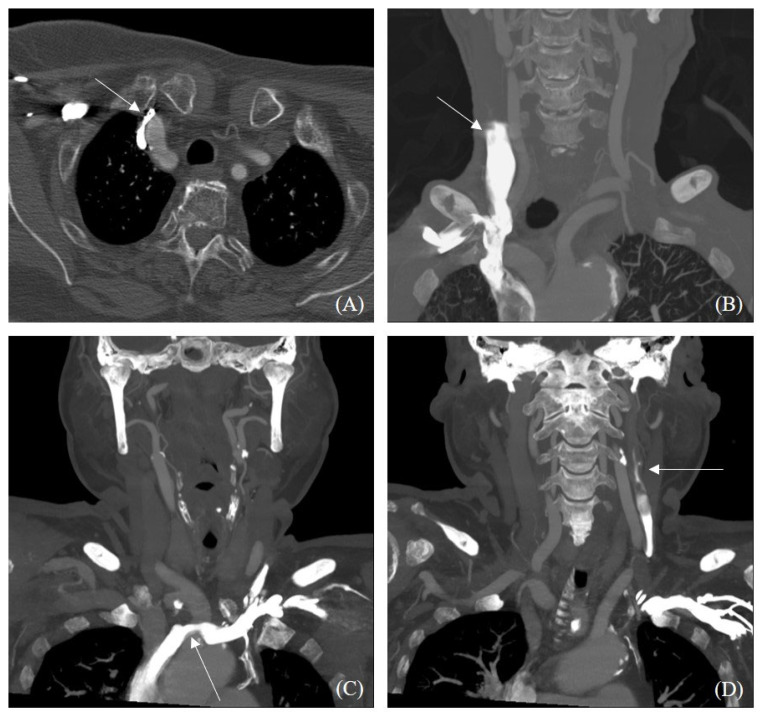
Venous reflux into internal jugular vein visible on CT angiography. Right brachiocephalic vein stenosis is observed due to tortuous course of brachiocephalic artery (A). Contrast reflux into ipsilateral internal jugular vein while administrating contrast media at peripheral vein at right forearm (B). Patient with left brachiocephalic vein stenosis with internal jugular vein reflux (C,D). The left brachiocephalic vein shows a longer and straighter course than the right brachiocephalic vein, and is more likely to be accompanied by stenosis between the aortic arch and sternum.
